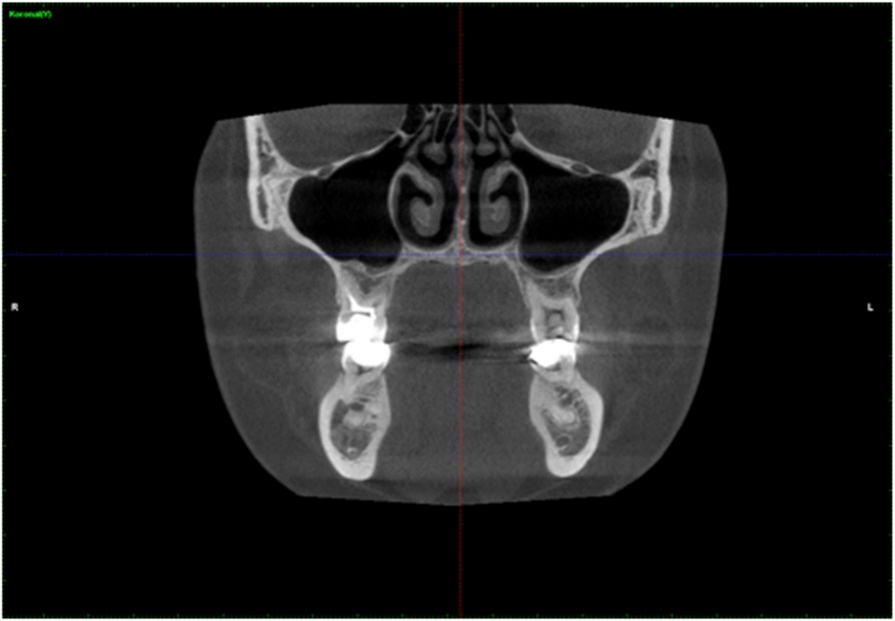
Making the nasal base parallel to the horizontal plane for standardization in CBCT coronal sections
